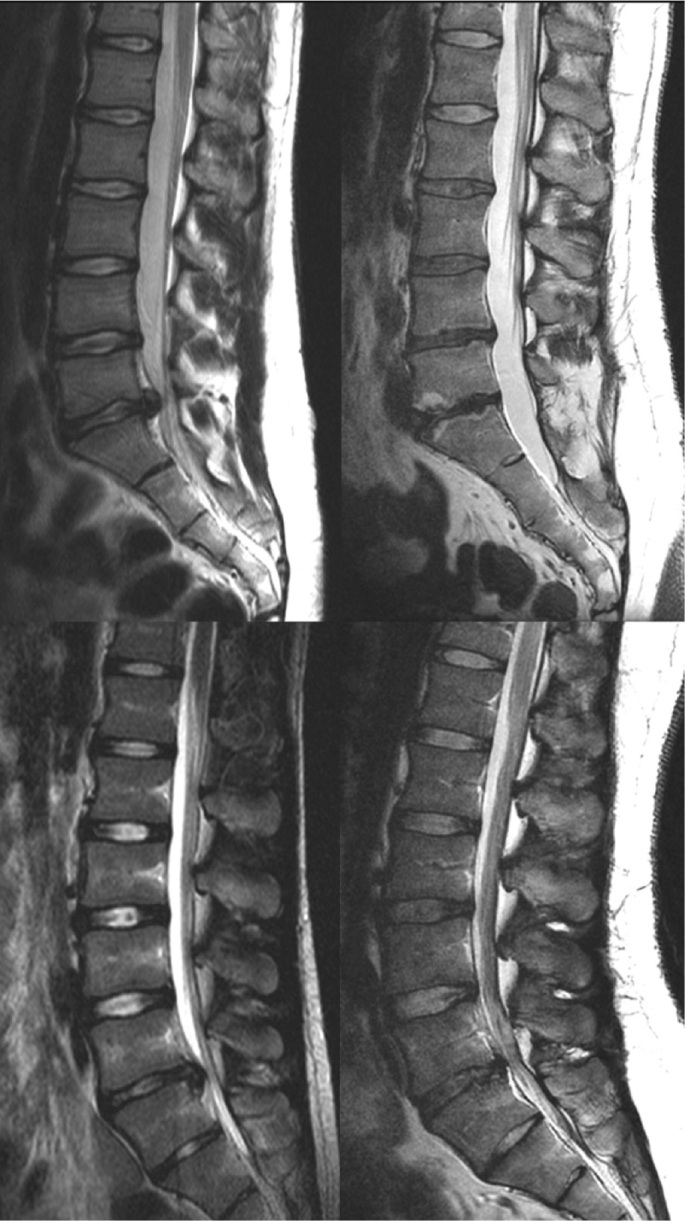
Baseline and follow-up MRI of a surgically treated individual (top) and a non-surgically treated individual (bottom) with disc herniations at level L5–S1. Baseline MRIs are shown on the left, and follow-up MRIs on the right. Degeneration according to Pfirrmann in the surgically treated individuals progressed from 1 to 3 at level L2–L3 and L3–L4, from 2 to 4 at L4–L5, and from 2 to 5 at L5–S1. Modic type 2 changes are seen at the L5–S1 level. In the non-surgically treated individuals, degeneration according to Pfirrmann progressed from 1 to 3 at L3–L4 and from 2 to 4 at L5–S1.
